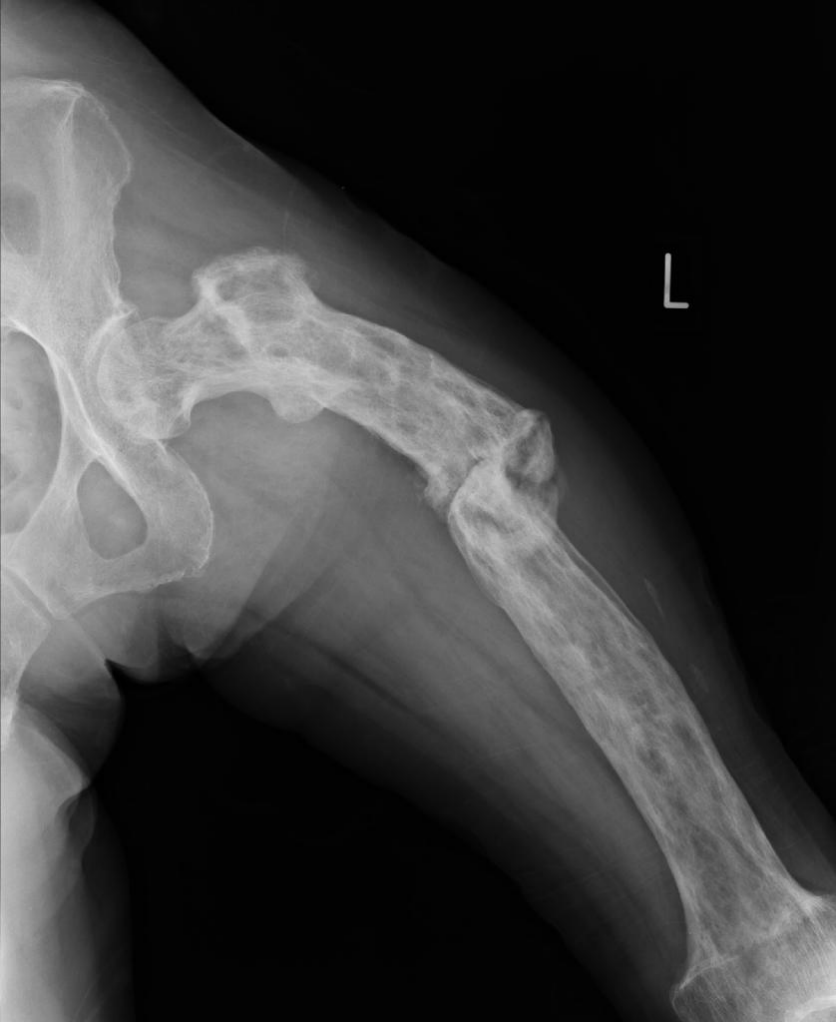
Anteroposterior radiographic view showing fracture healing 6 months after application of the functional brace.
